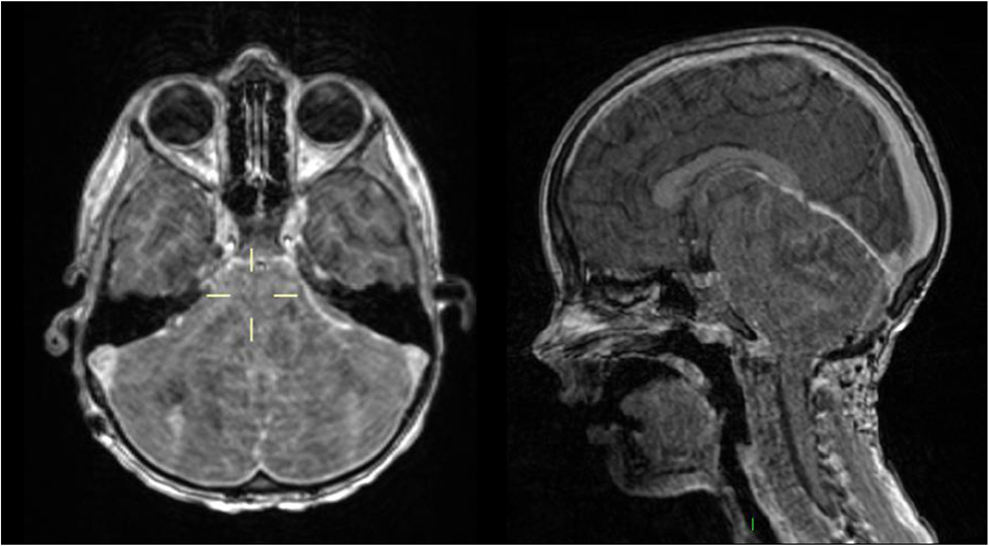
MRI 41 days post-resection
